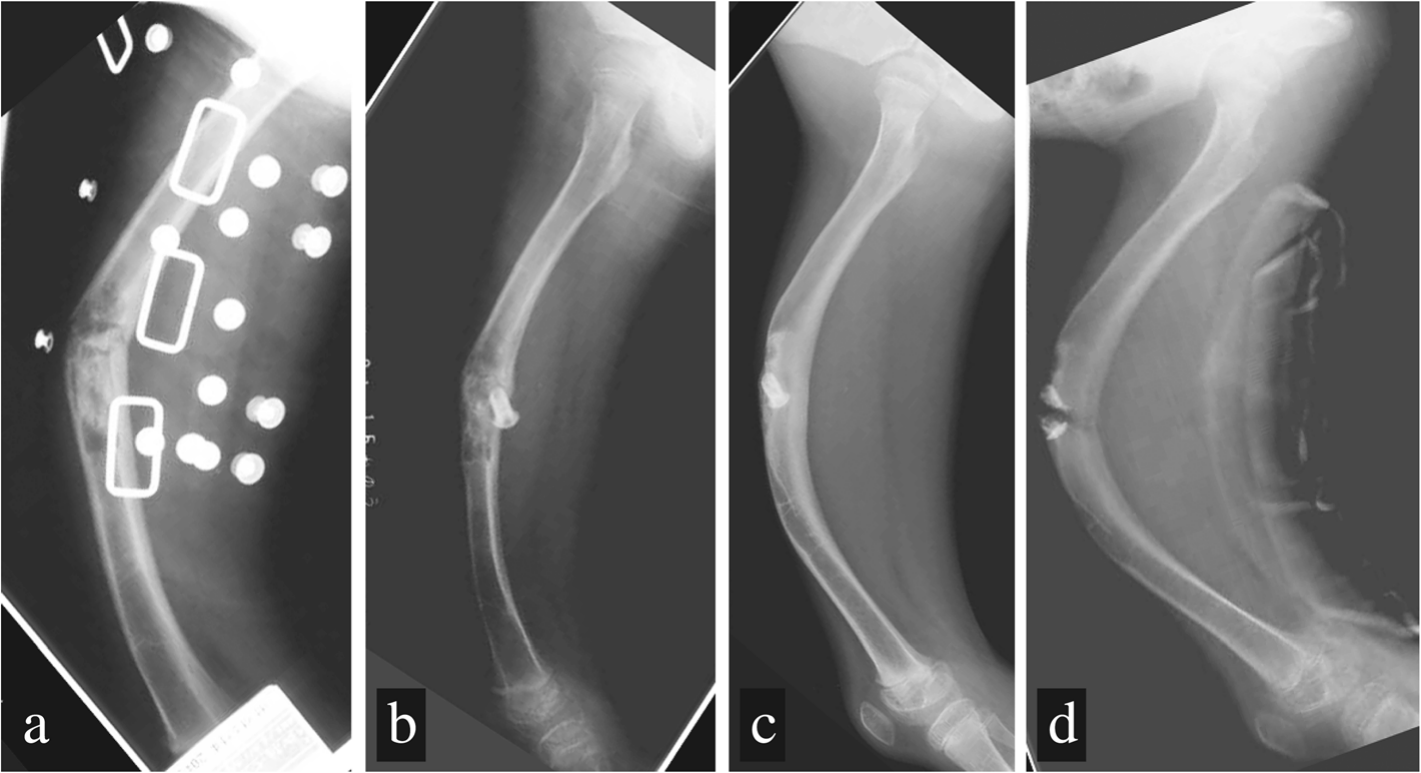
Right femur lateral radiographs. a Third fracture at age 6 years, 4 months. b After the bone fracture had healed with the cannulated screw inserted. c At age 9 years, 3 months, the patient wore a functional brace, but the bowing deformation gradually worsened. d At age 9 years, 6 months, femur fracture developed at the cannulated screw
